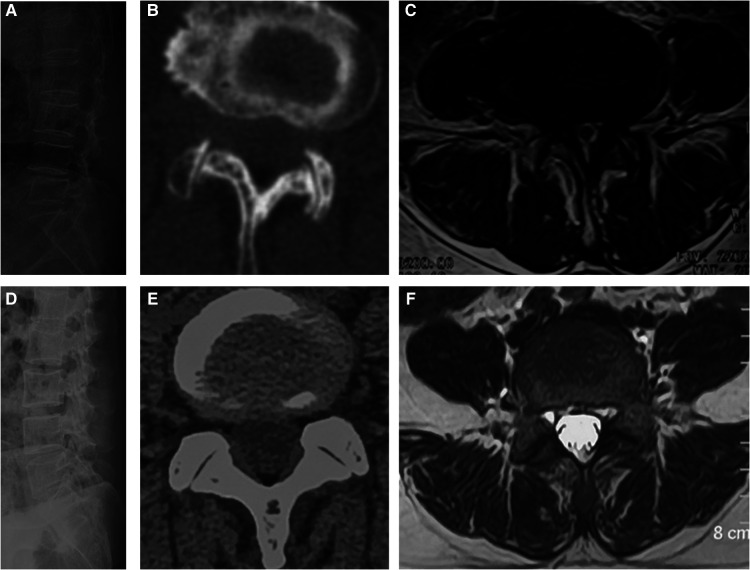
(A, B and C) are x-ray, CT and MRI of DLS patients, respectively. (D, E and F) are x-ray, CT and MRI of Normal subjects respectively.
